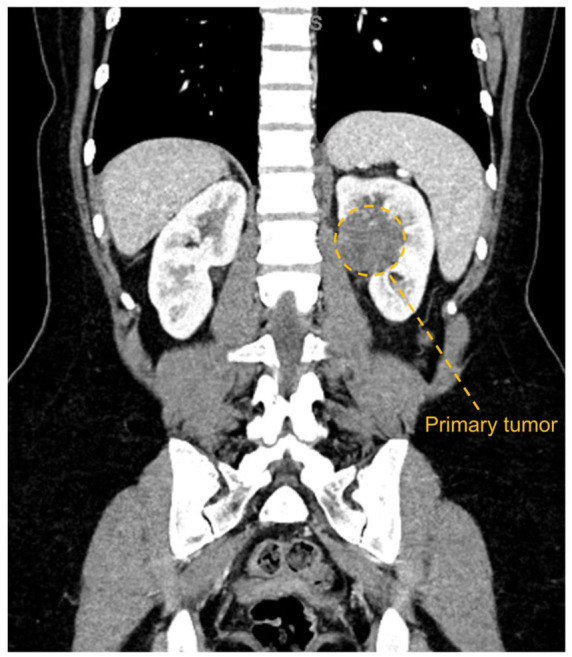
Coronal CT view of the primary left kidney tumor mass prior to nephrectomy.
